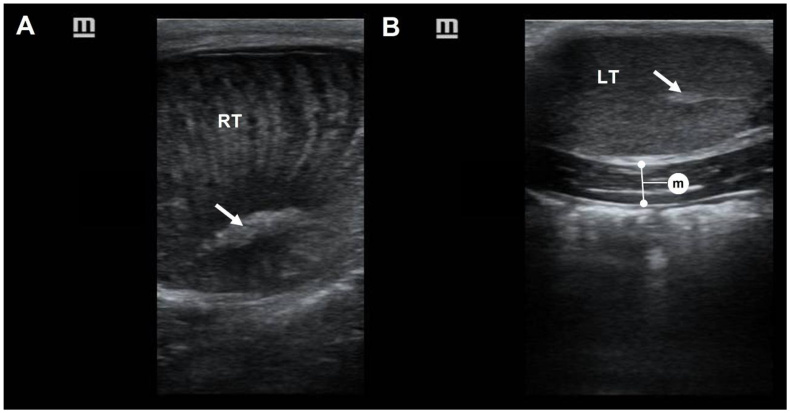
Ultrasonographic findings of the testes of a collared peccary (*Pecari tajacu*) with unilateral cryptorchidism. (A) Right testis, located within the scrotal sac, showing preserved contour, shape, and mediastinal line, and a mixed echotexture, with echogenic lines alternating with hypoechogenic areas distributed throughout the testicular parenchyma and converging toward the hyperechogenic mediastinum; (B) Left testis, located in the inguinal region, in the subcutaneous tissue, showing preserved contour and shape, decreased echogenicity, and homogeneous echotexture, with a central hyperechogenic mediastinum. Legend: right testis (RT); left testis (LT); testicular mediastinum (arrow); abdominal wall muscles (m).
